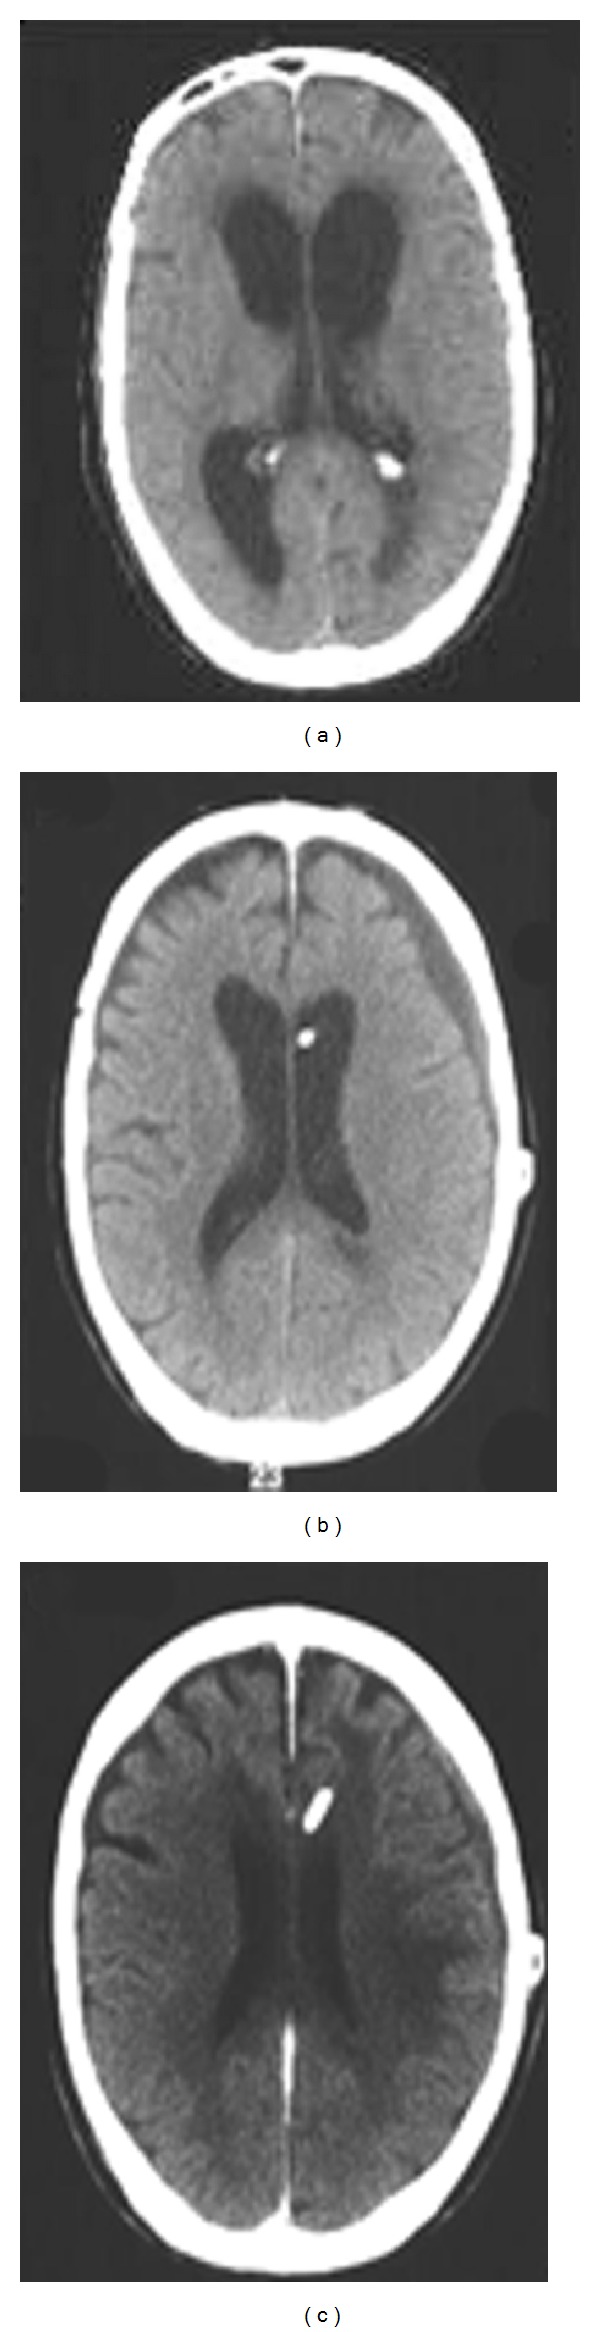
(a) Preoperative CT scan showing enlarged ventricular system. (b) CT scan four weeks after insertion of a shunt, subdural hygroma can be observed (c) CT scan 6 weeks after the adjustment of the opening pressure. The hygroma had reabsorbed.
